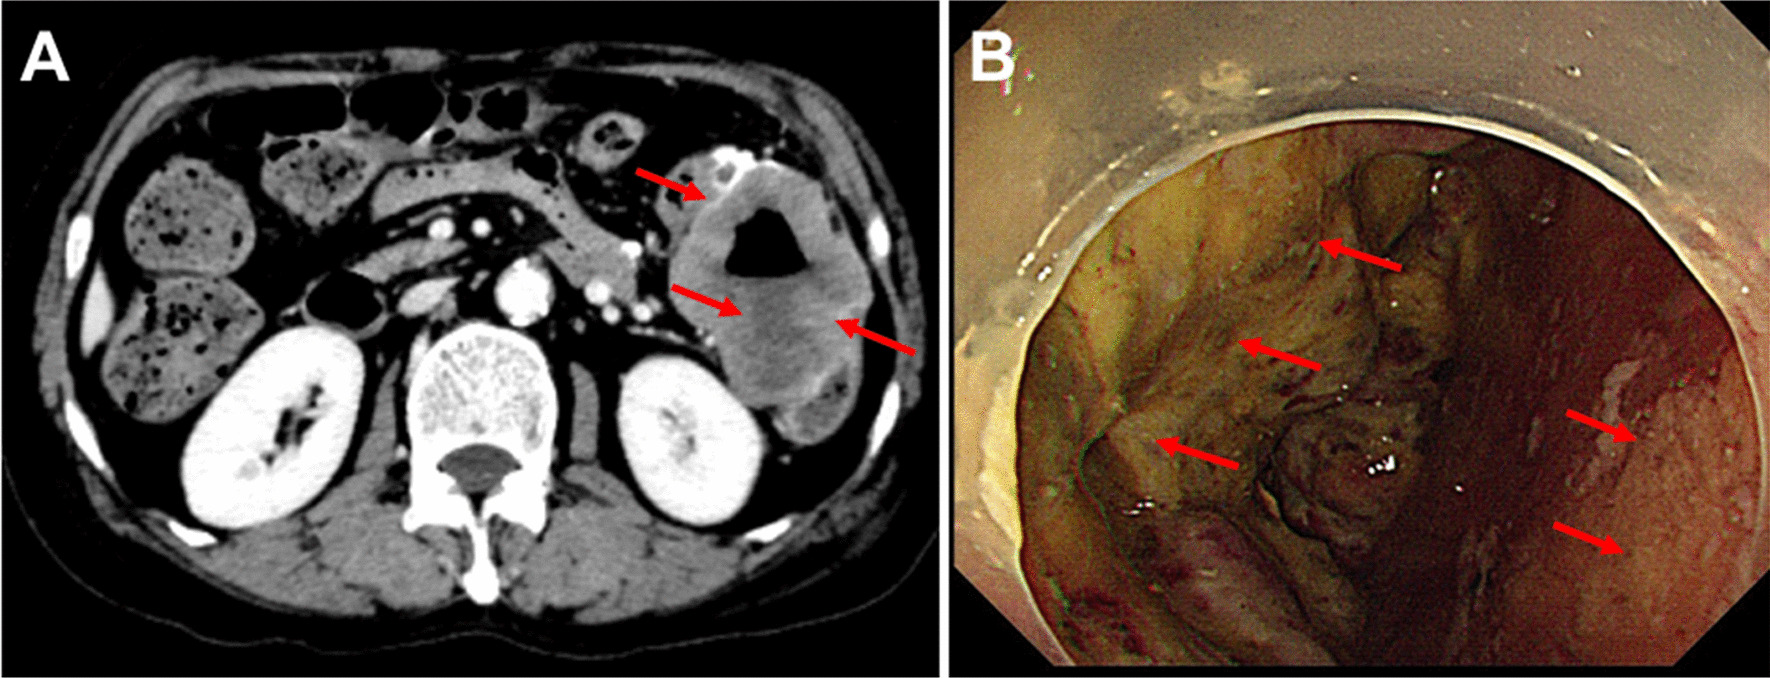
Abdominal CT and electric colonoscopy examinations. A Abdominal CT examination indicates the tumor (red arrows) in the descending colon. B Colonoscopy shows the ulcerative tumor (red arrows), which has invaded the descending colon wall
